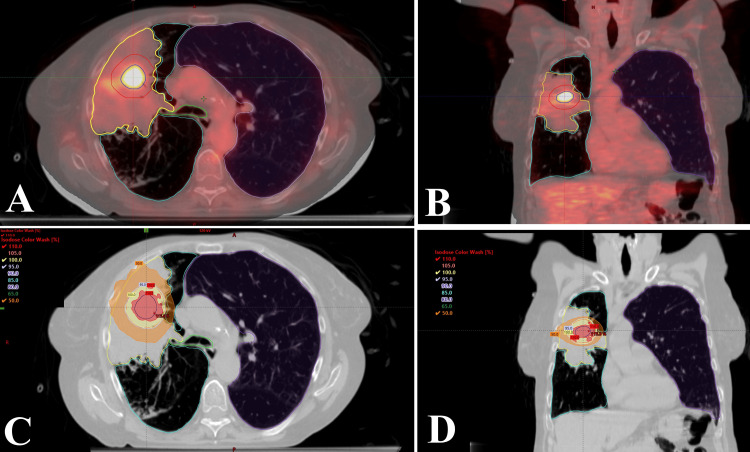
Treatment planning (A) Axial and coronal (B) views of the delineated gross tumor volume (blue) and planning target volume (red) on the fused positron emission tomography-computed tomography scans. (C) Axial and (D) coronal views of the radiation treatment plan showing the dose coverage of the gross tumor volume and planning target volume. Also shown are the region of atelectasis (yellow), distal bronchus (green), ipsilateral (cyan) and contralateral lungs (purple).
